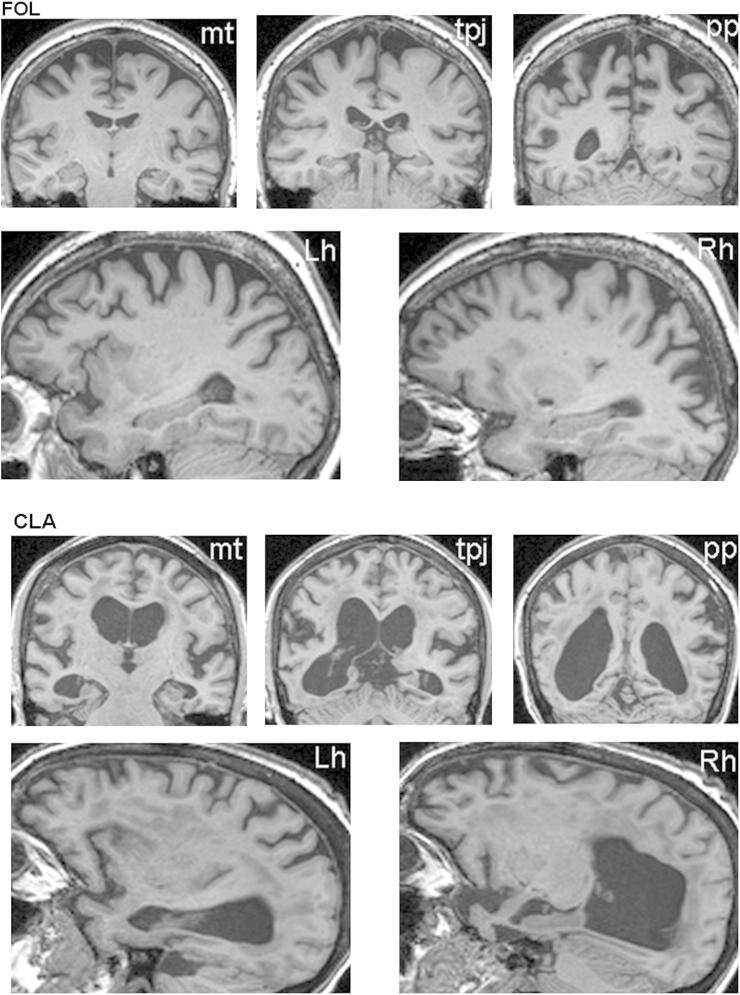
Neuroanatomical features in FOL and CLA. Representative brain MRI sections for each patient show the distribution of atrophy in each case. Coronal sections (upper panels in each case) are in the plane of the mid-temporal lobe (mt), temporo-parietal junction (tpj) and posterior parietal lobe (pp), respectively; the left hemisphere is shown on the right for all coronal sections. Sagittal sections (lower panels in each case) are through the left (Lh) and right (Rh) cerebral hemispheres.
